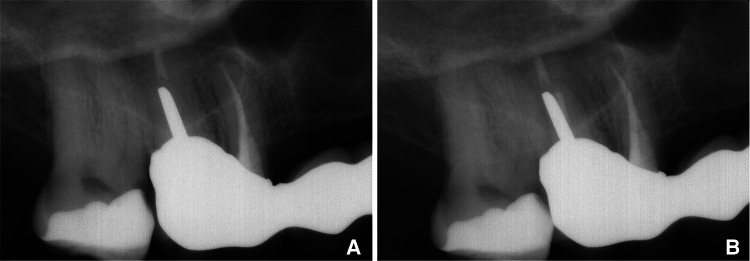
Comparative radiographs showcasing the 6-month follow-up post-treatment. (A) Alongside the 9-year follow-up (B), Emphasizing the sustained treatment effectiveness and stability over time.
